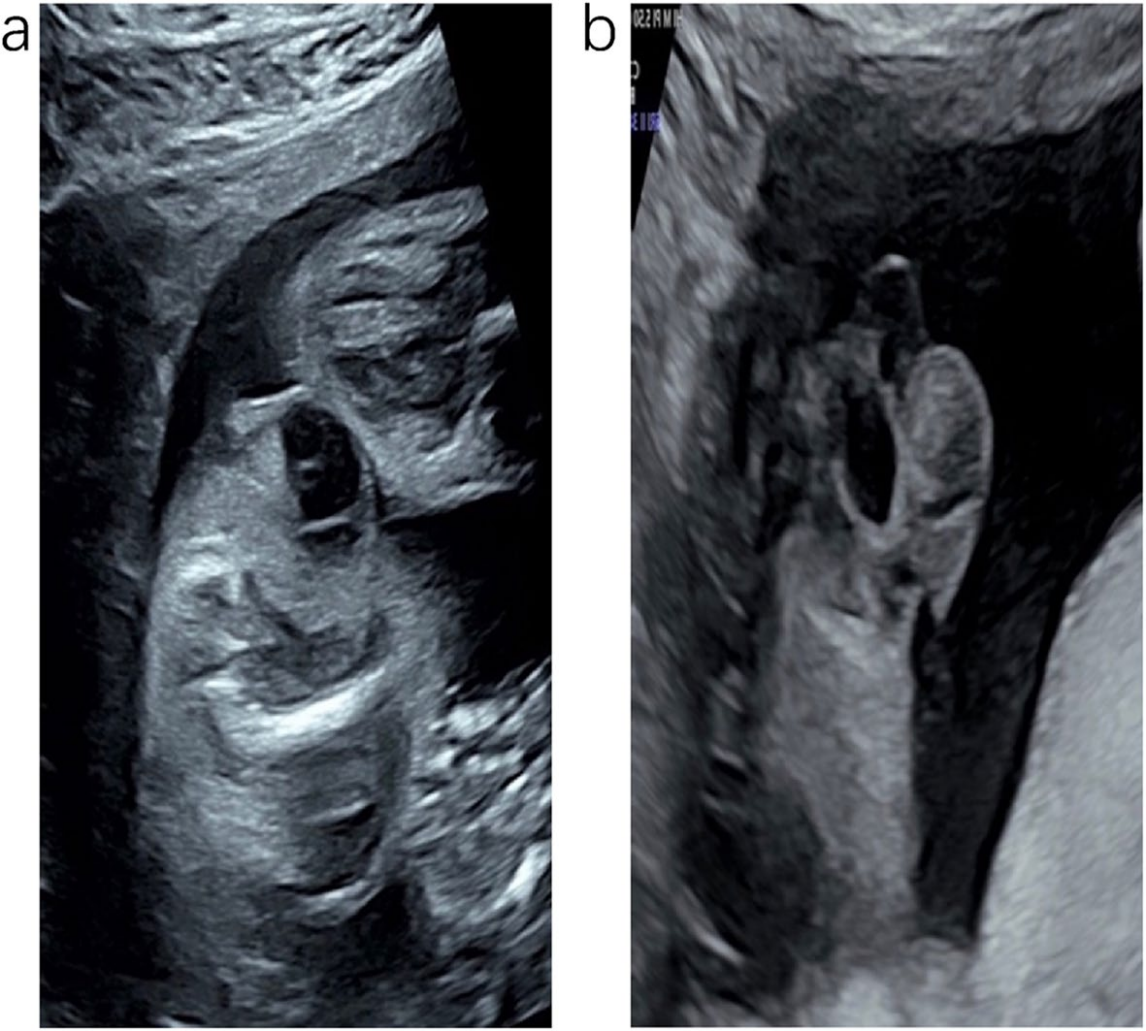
Prenatal ultrasound (a) Two-dimensional ultrasound showing a cystic mass in the perineum at 24 gestational weeks. b A solid component began to appear in the cystic mass at 27 gestational weeks
